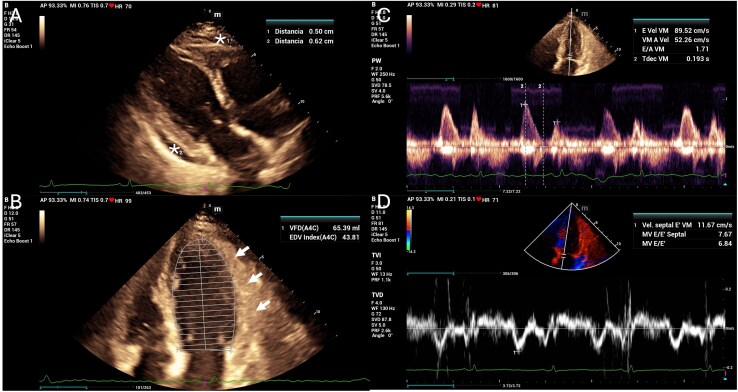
Transthoracic echocardiogram. *(A)* Right parasternal long-axis image showing pericardial effusion (asterisks) and normal-sized left cavities. *(B)* Apical four-chamber image showing pericardial effusion with areas of pericardial hyper-refringence (white arrows). *(C and D)* Pulsed and tissue Doppler showing a preserved E/A and septal ratio without flow variability.
